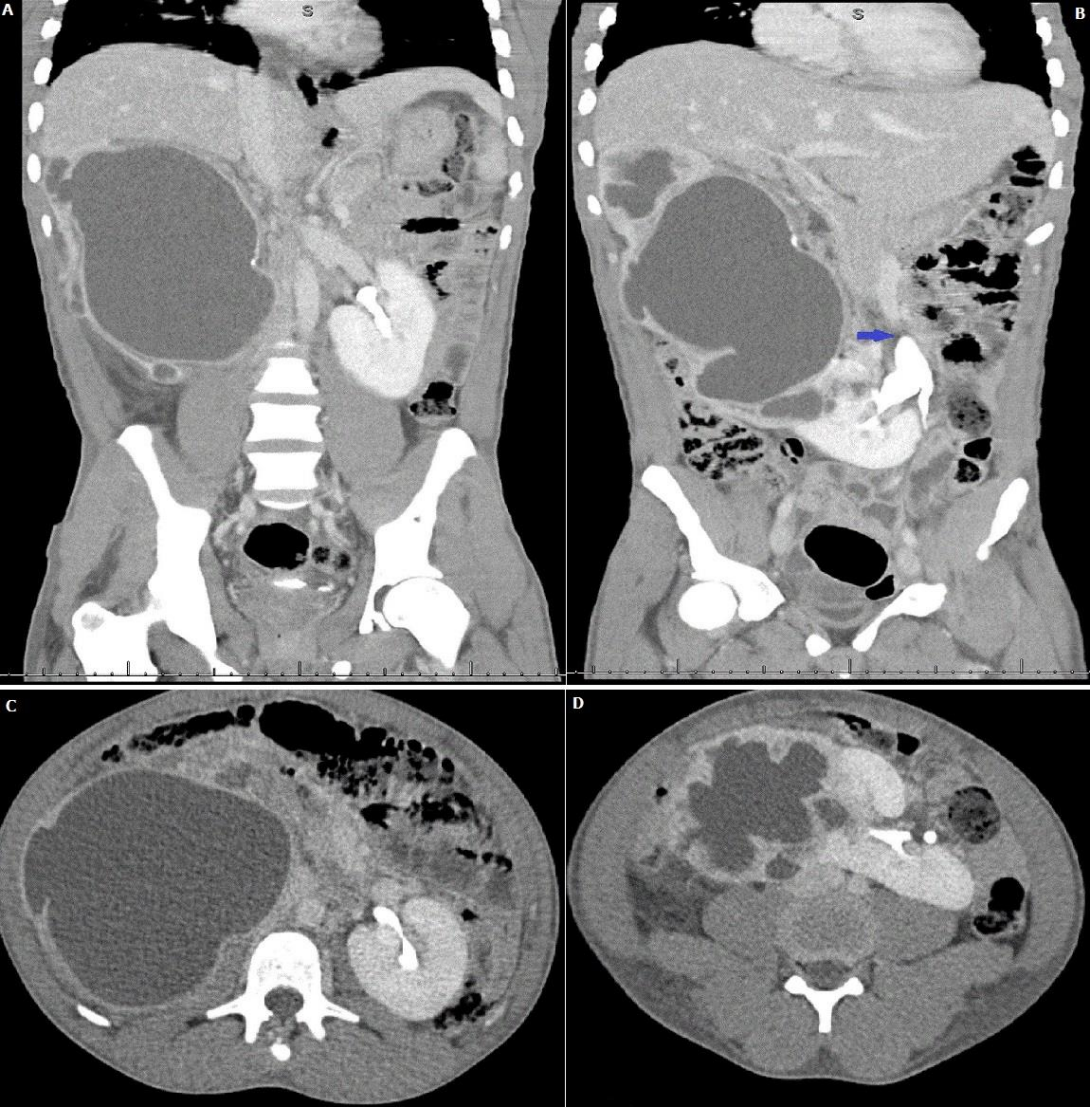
CT-IVP coronal (A, B), axial (C, D) images of the horseshoe kidney, the blue arrow labels the PUJ obstruction
